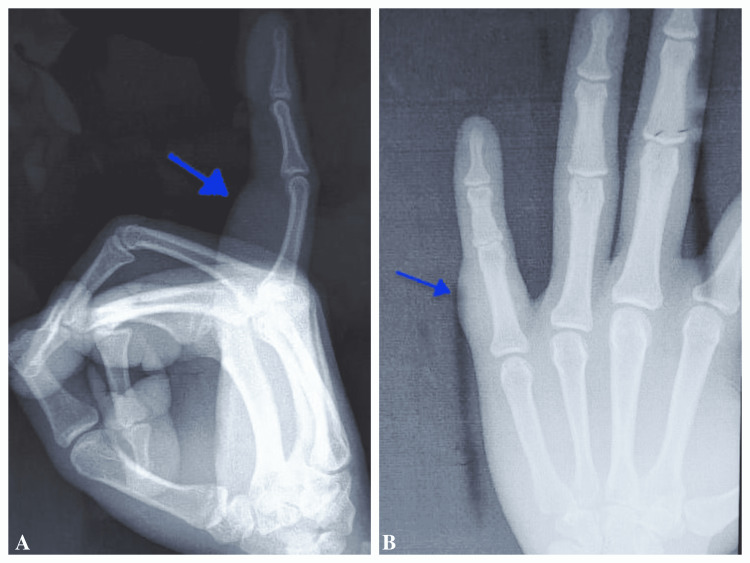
X-ray showing subcutaneous mass swelling in lateral view (A) and anteroposterior view (B)
